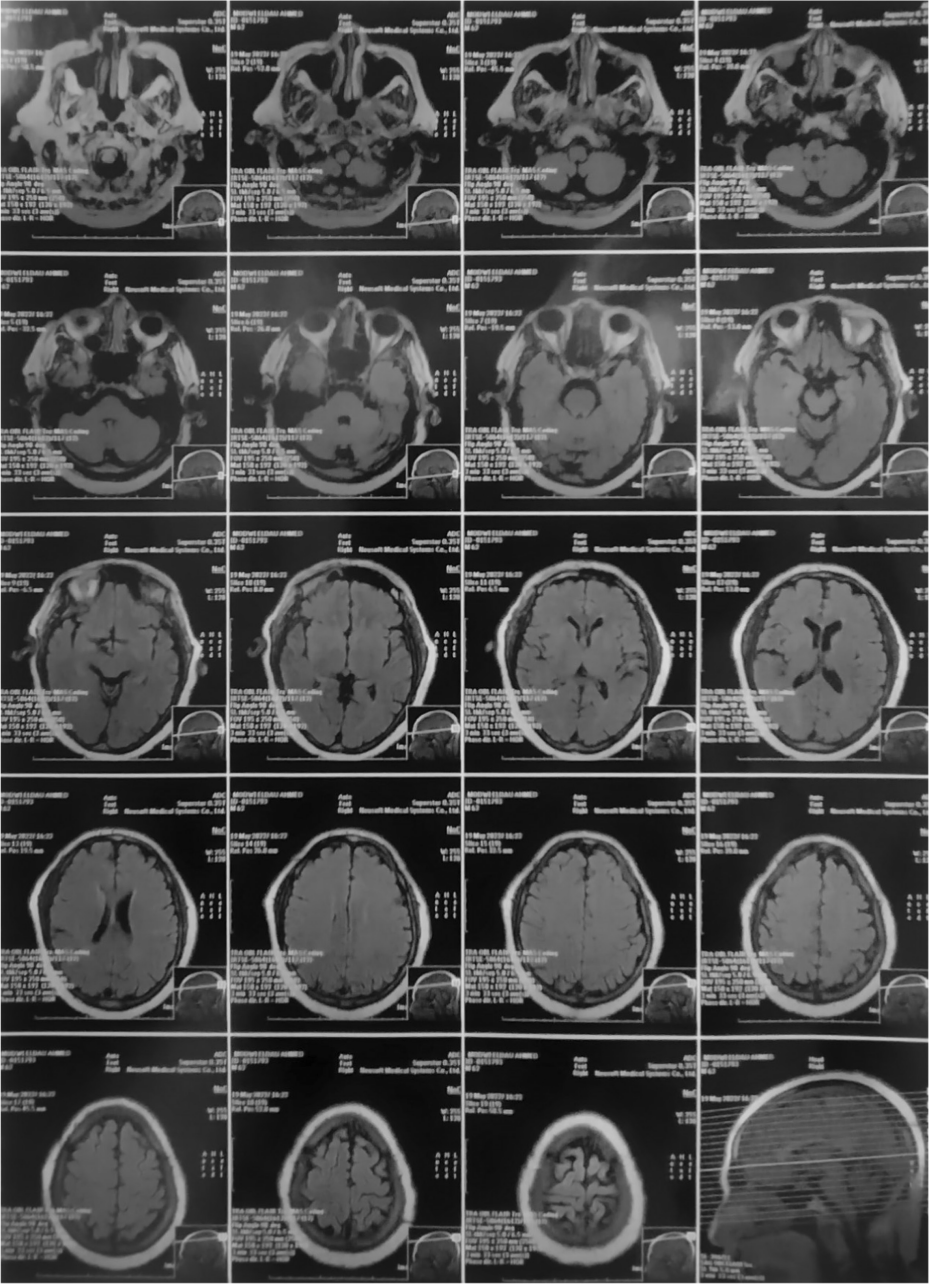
A Magnetic Resonance Image: The MRI shows the absence of lesions and structural abnormalities
